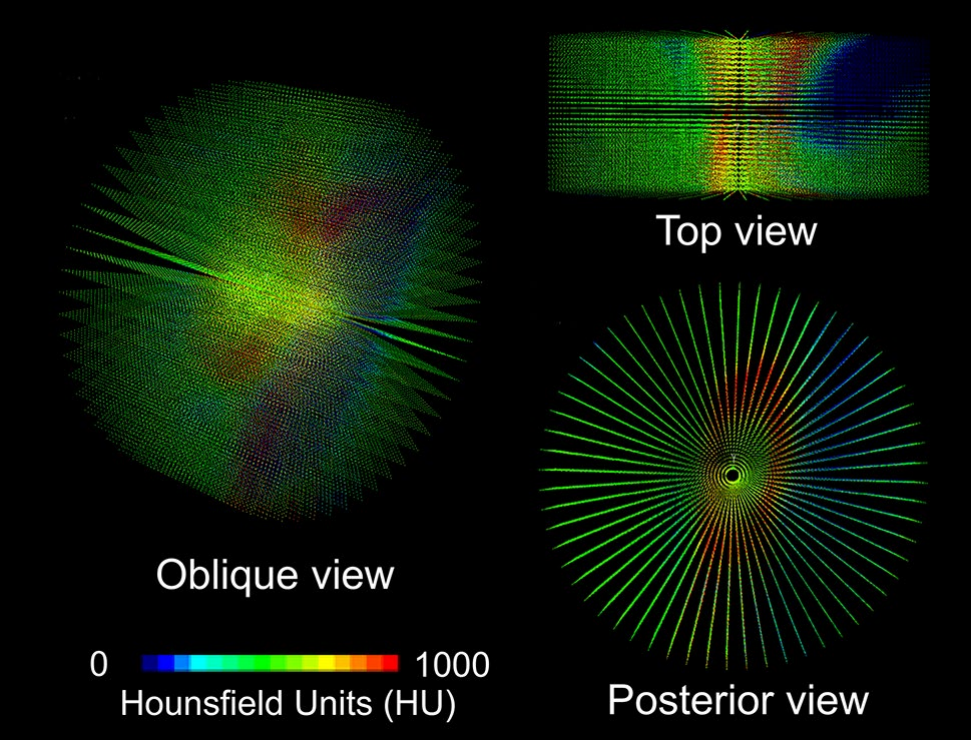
3D attenuation distribution in a cylindrical space centered about the pedicle axis obtained directly from a CT DICOM dataset. The 3D geometry of the pedicle cortex can be inferred from the high HU values with reddish color hues.
